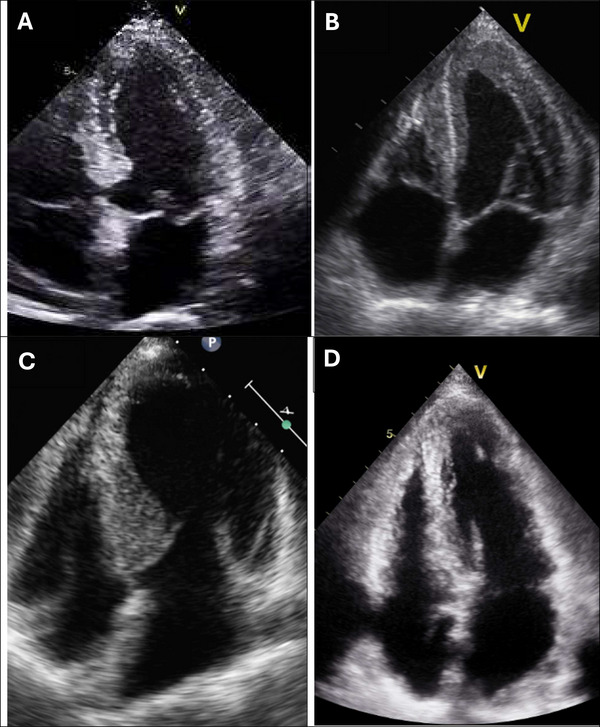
Echocardiographic variants of HCM. (A) Asymmetric septal hypertrophy with a reverse curvature of the interventricular septum. (B) Symmetric septal hypertrophy with a neutral septal contour. (C) Sigmoid‐shaped septal hypertrophy localized to the basal portion of the septum. (D) Apical hypertrophy limited to the apex, with normal wall thickness in the basal segments.
